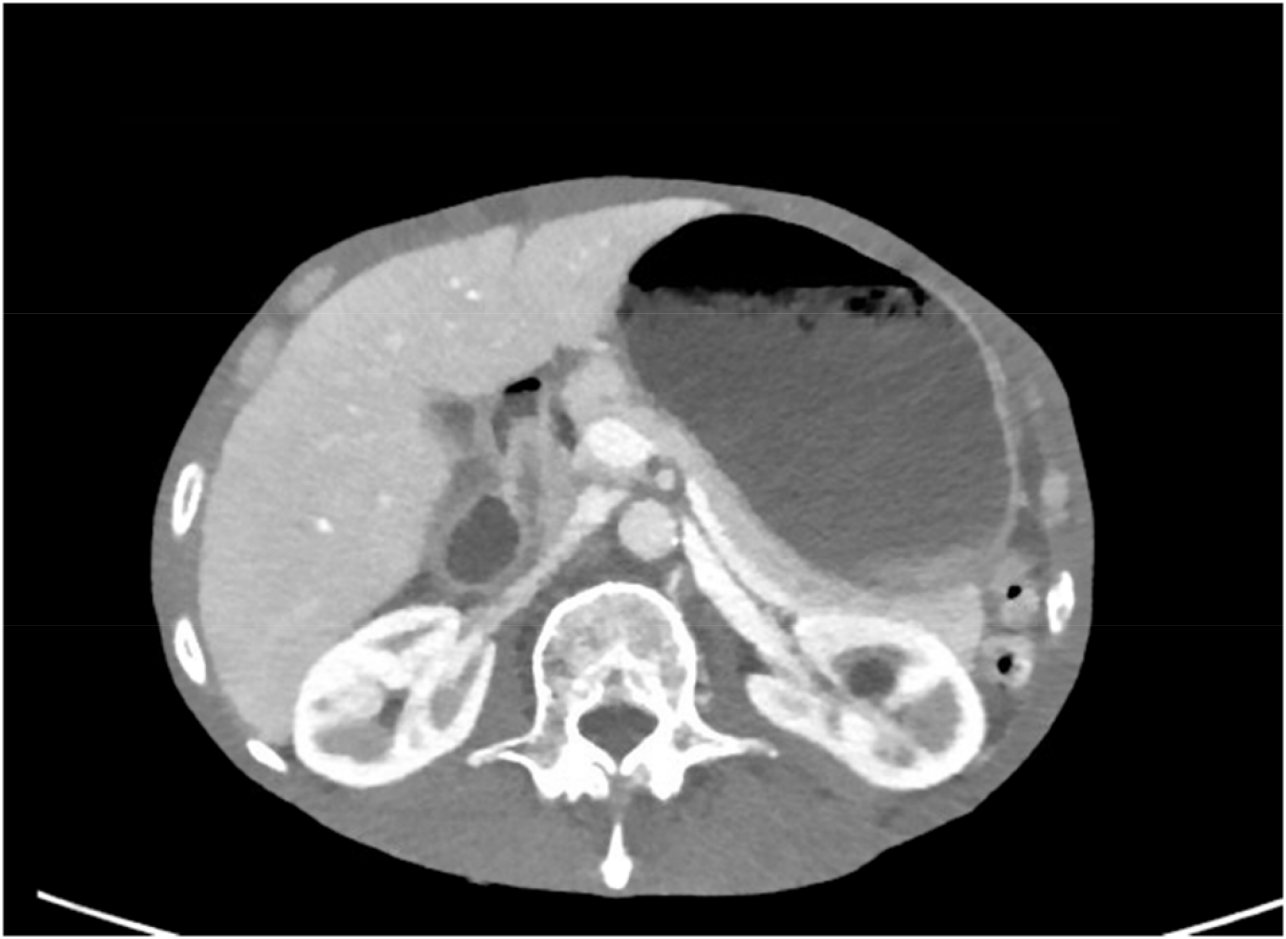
CT imaging of gastric distention.
